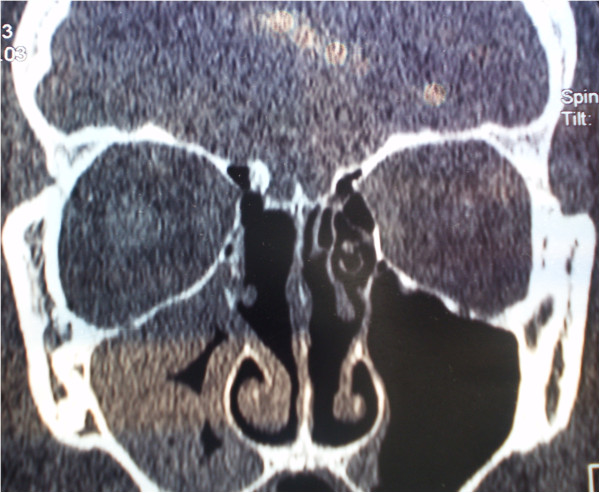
Coronal multislice computed tomography one year postoperatively.
